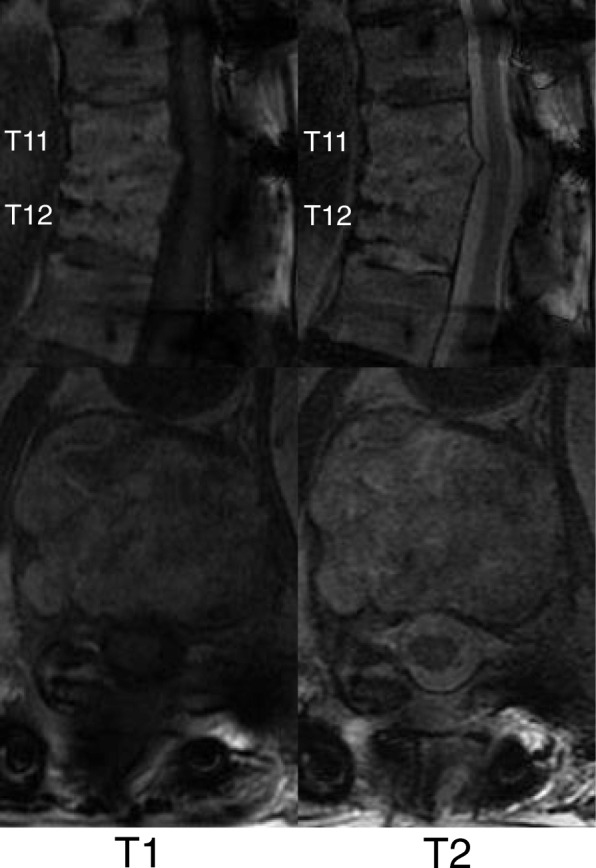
Magnetic resonance imaging revealed no evidence of increased signal intensity at T11–T12 vertebral bodies and severe cord compression and epidural abscess at T11–T12
